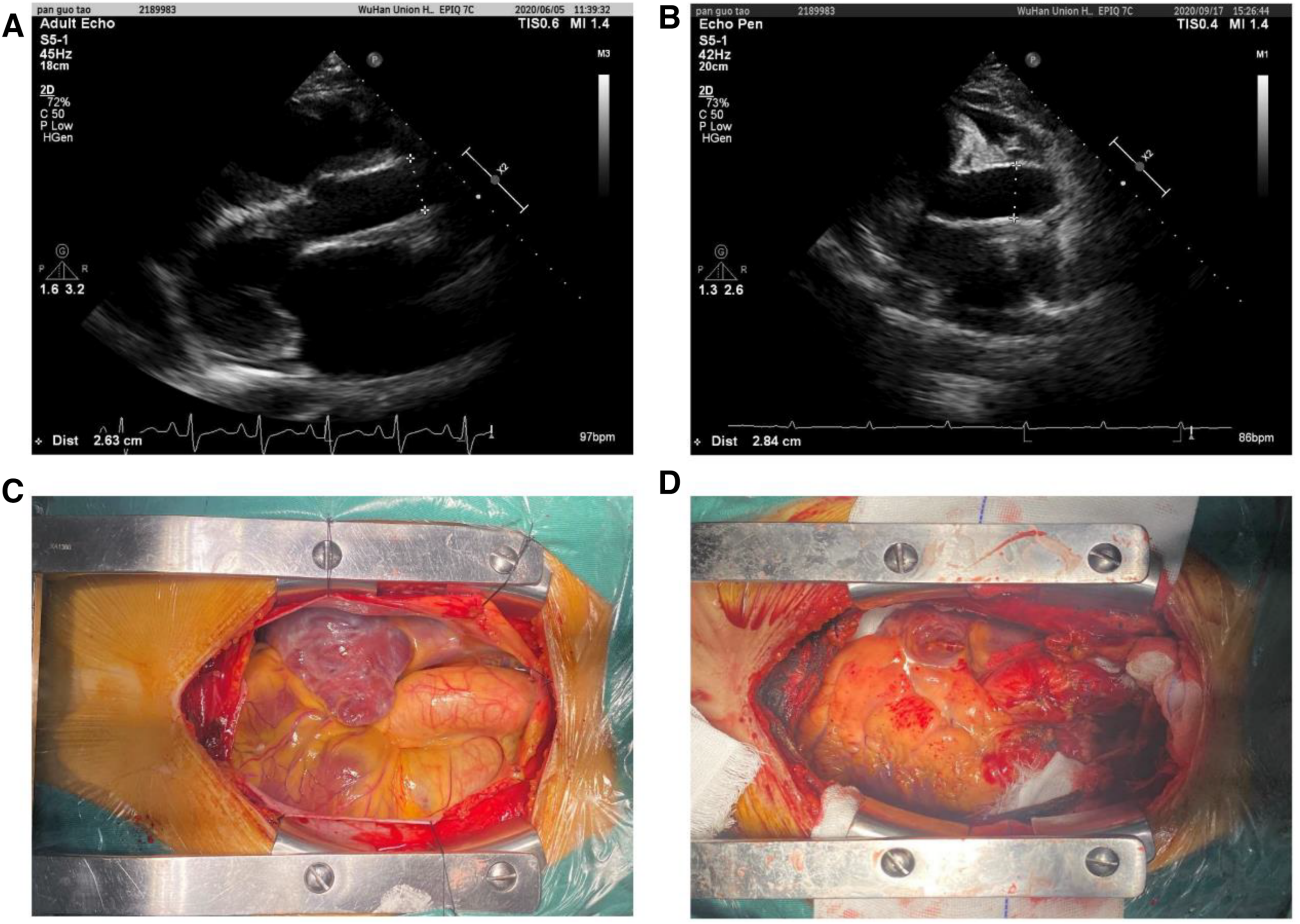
Ascending aortic diameters measured using the leading edge-to-leading edge convention in preoperative (A, recipient) and postoperative (B, donor) by two-dimensional echocardiography. Intraoperative AAo image before heart removal (C) and AAo image after donor heart implantation (D).
